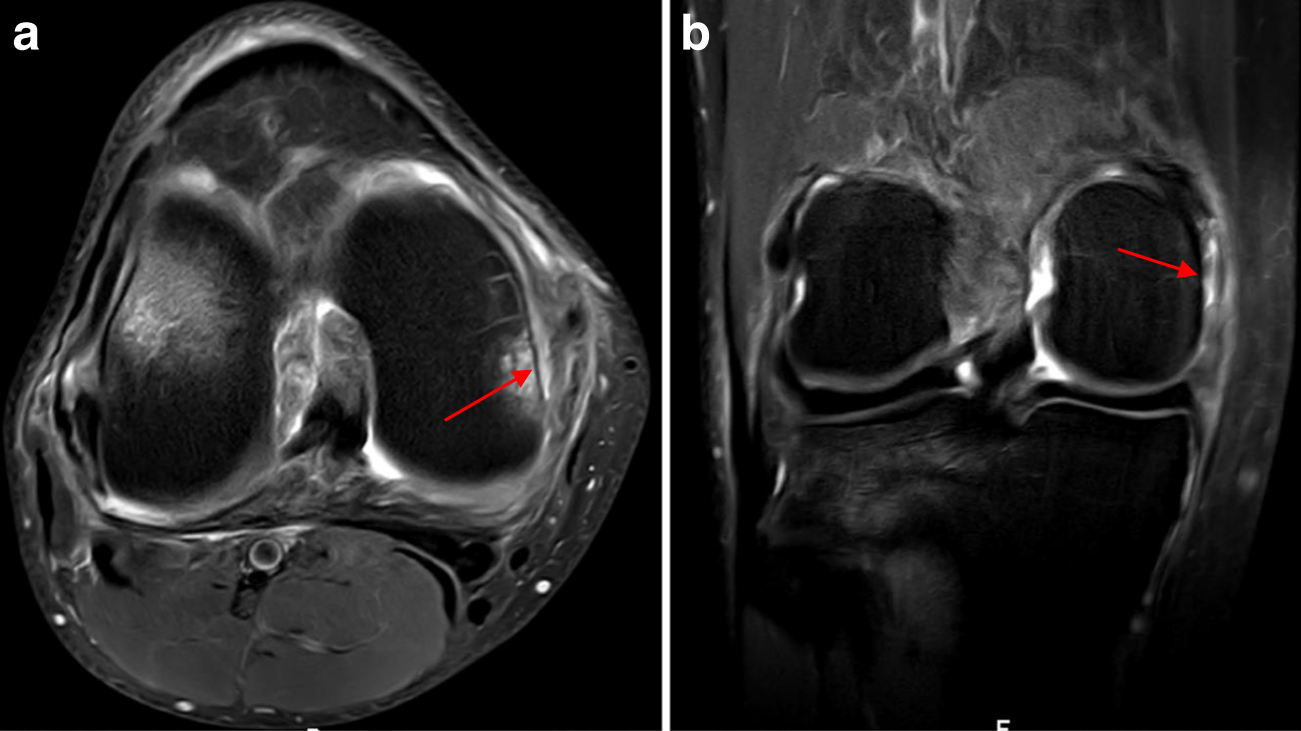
Posterior oblique ligament injury (arrows) on axial sequence above level of the joint line (a) and on coronal sequence posterior to the medial collateral ligament (b) on magnetic resonance imaging sequences (right knee)
